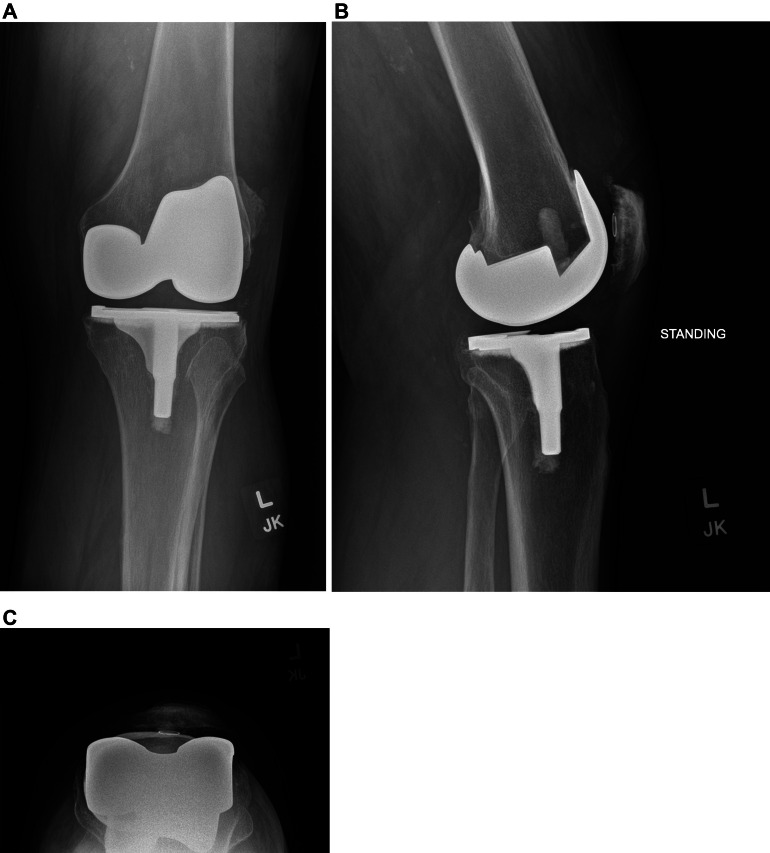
(A), (B), and (C). Frontal, lateral, and tangential patellar radiographs show a left total knee arthroplasty with well-seated components.
